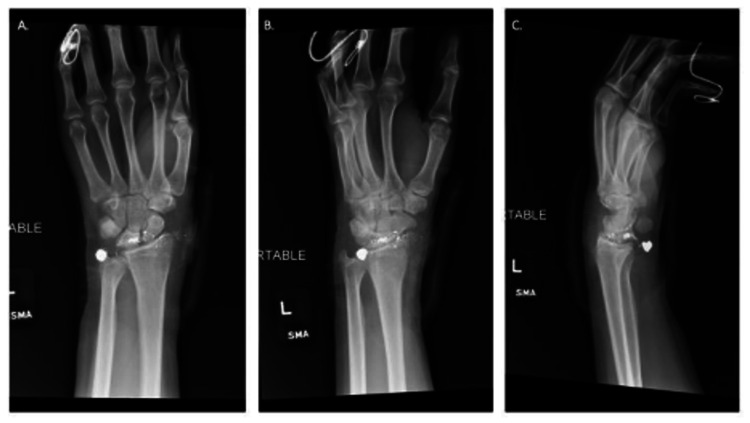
Preoperative plain radiographs. Anteroposterior and lateral X-Ray images (A-C).
